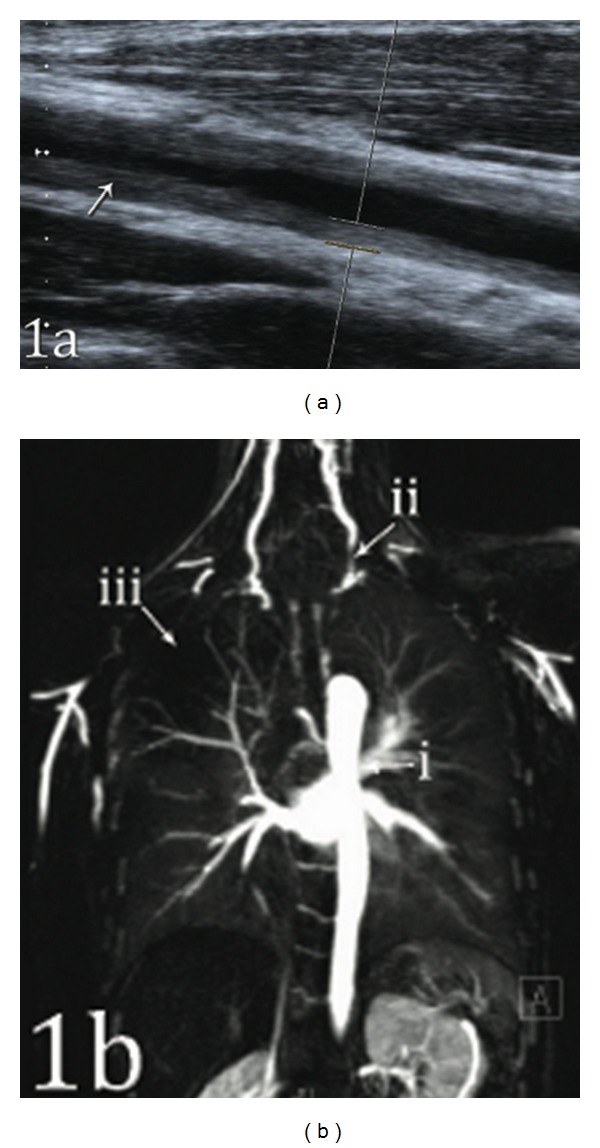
(a) Doppler ultrasound of the left common carotid artery demonstrating marked wall thickening with subsequent loss of lumen inkeeping with stenosis (arrow). (b) MR angiothorax/neck exhibiting widespread pathological changes in the arterial vessels: narrowing of the thoracic aorta (i), stenosis of the left carotid artery (ii), and bilateral occlusion of the subclavian arteries (iii), with collateral distal filling via the intercostal arteries.
